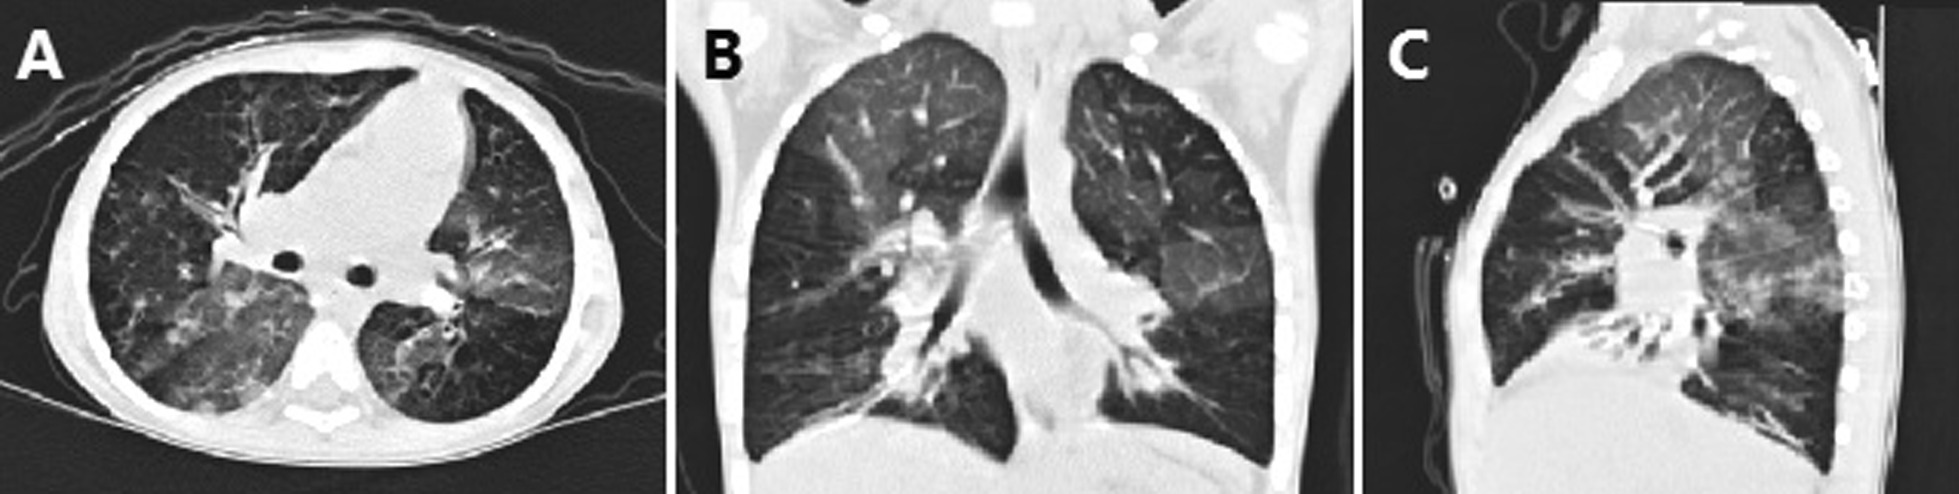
High-resolution computed tomography (CT) scan of the chest revealing small airway obstruction lesions with double pneumonia, insufficiency of the right middle and left lower lung segments, and local bronchiectasis in both lungs on first hospitalization
